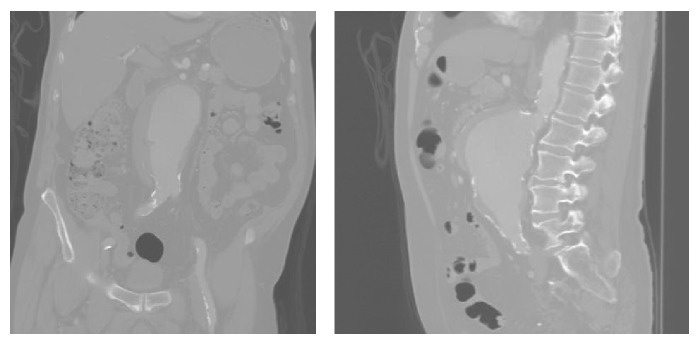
Juxtarenal AAA on CT scan.
